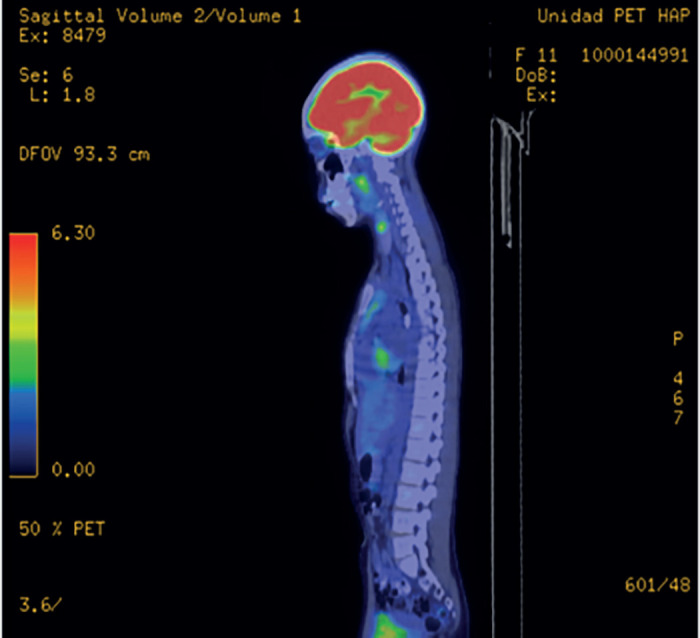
Sagittal PET CT. Source: Clinical record.
